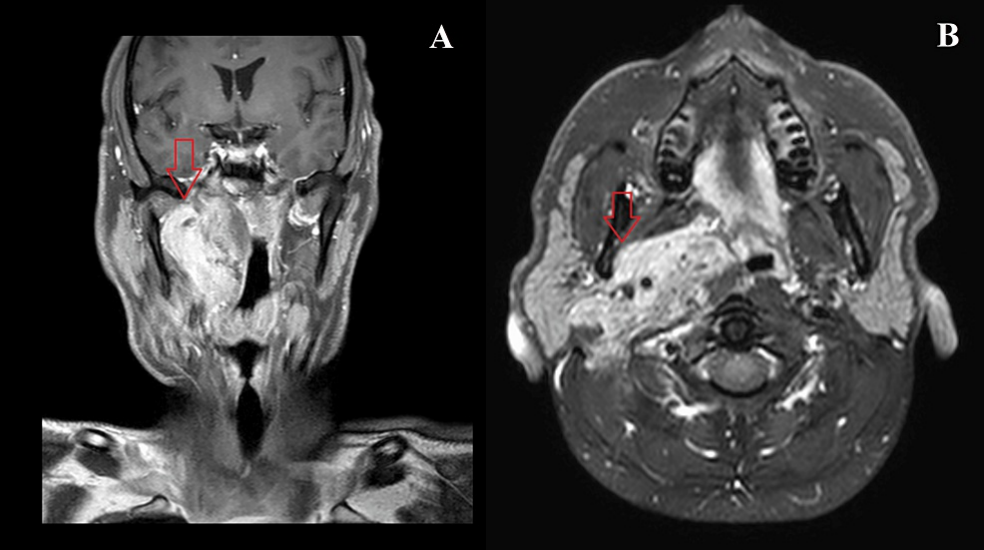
Magnetic resonance imaging of face and neck showing pretreatment images of the locally advanced tumor on the right side as indicated by the red arrows (A) Coronal view. (B) Axial view.
